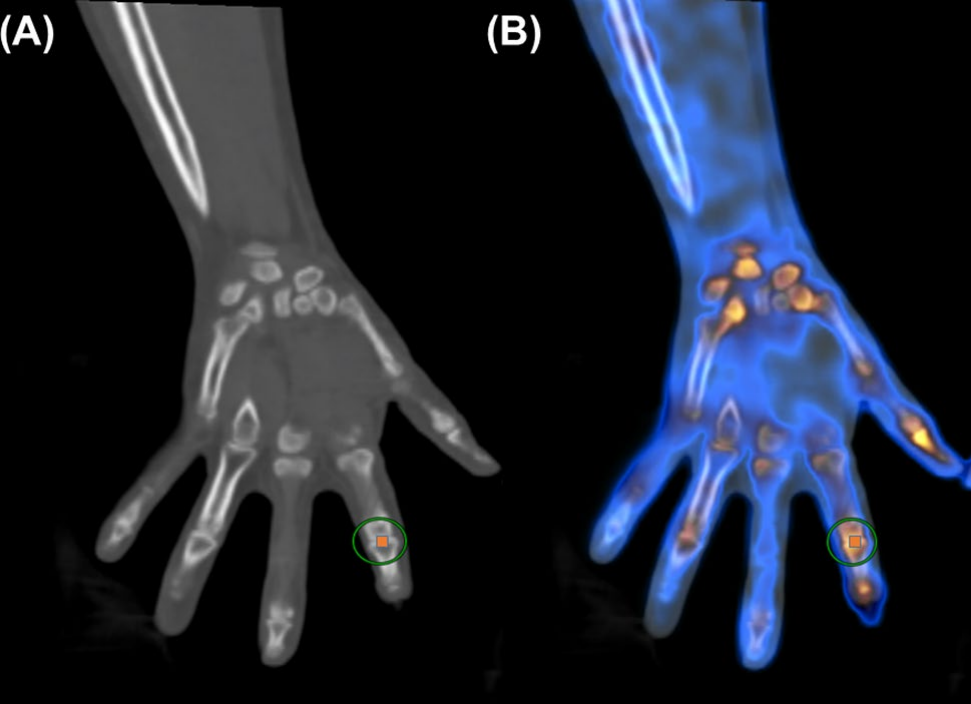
(A) CT and (B) SPECT/CT images demonstrate how the SUV was measured at the hand joint. The SUVmax was derived from a spherical VOI drawn over the periarticular region, placing the joint space at the center of the VOI.
